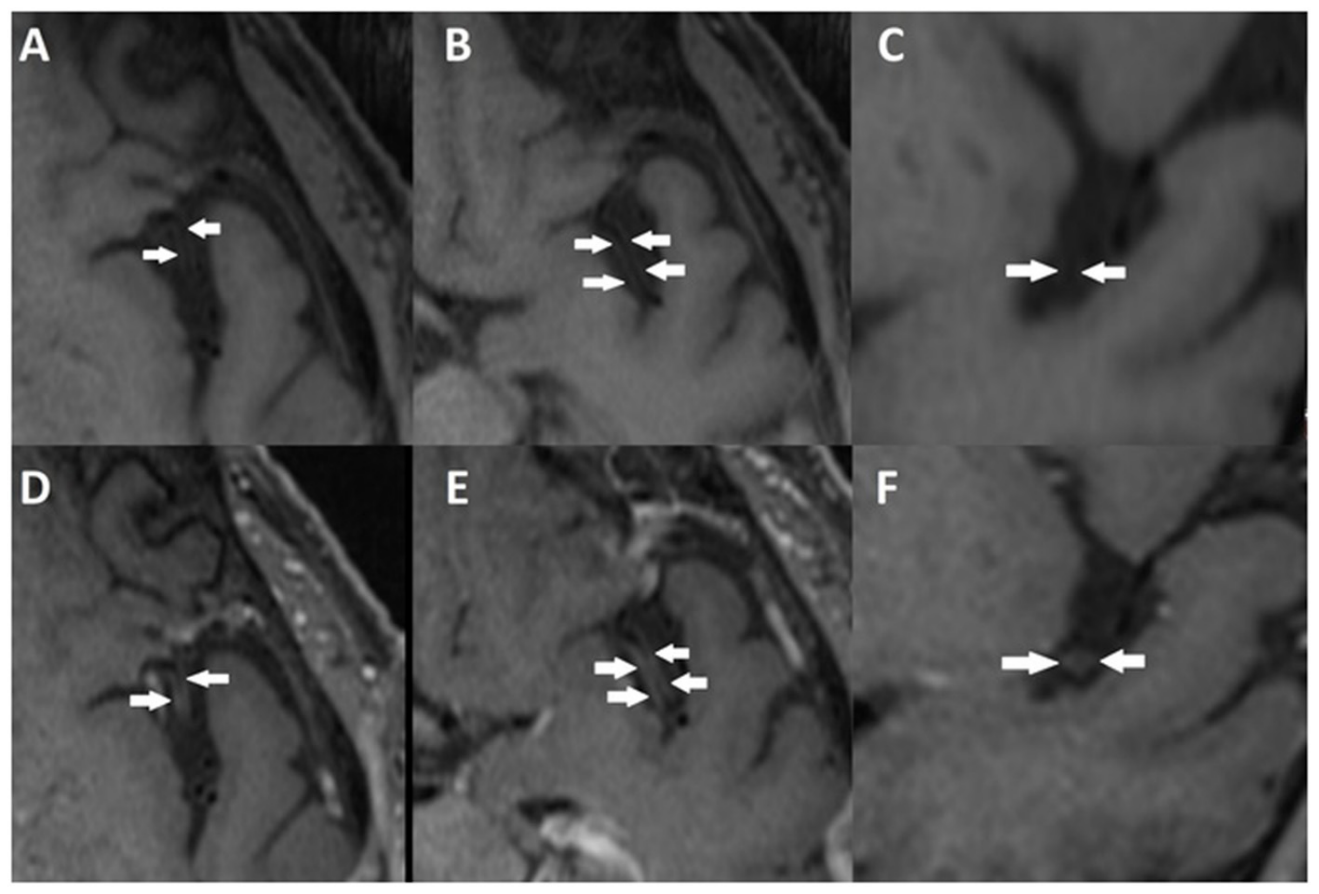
Vessel wall imaging (VWI-MR) of the brain of another patient who experienced post COVID-19 symptoms. Axial (A, B) and coronal (C) precontrast T1-weighted images, and contrast enhanced axial (D, E) and coronal (F) T1-weighted images, show segmental concentric wall thickening and enhancement of M2 left middle cerebral artery segments (*arrows*) consistent with cerebral vasculitis.
